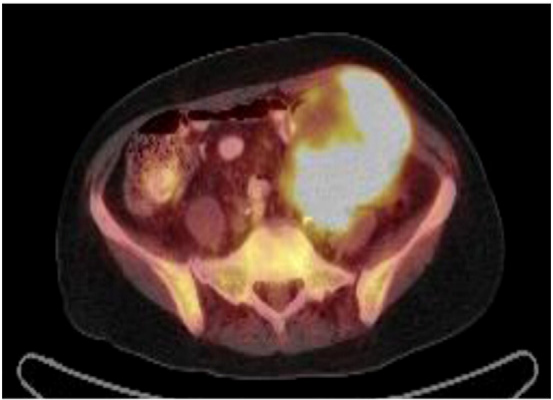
Fused PET/CT coronal image of the large hypermetabolic pelvic mass.
